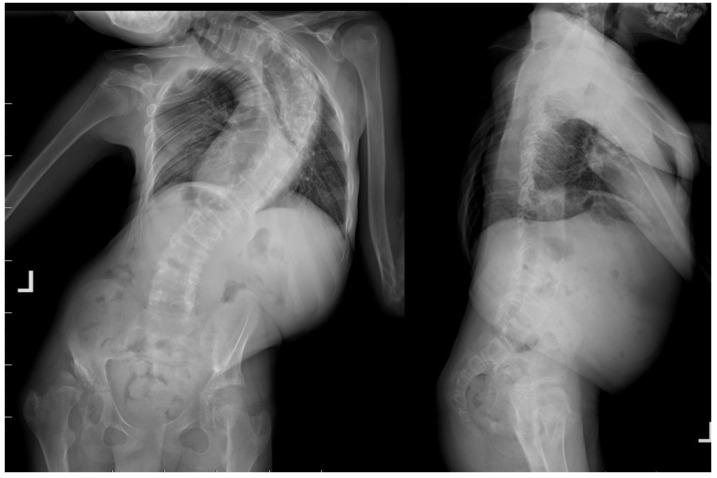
Radiological standing X-rays AP and LAT of 14-year-old immature patient with Costello syndrome, severe spinal deformity measured of 130 degrees.
